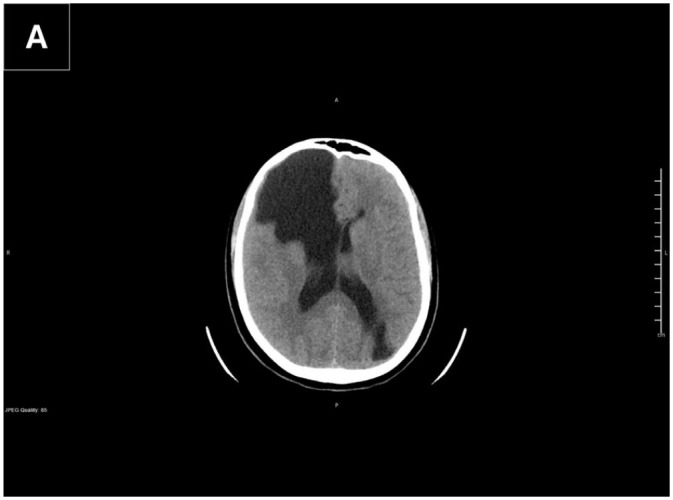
(a) Axial non-contrast CT of the head demonstrates a large porencephalic cyst in the right frontal lobe communicating with the right lateral ventricle, associated with ex vacuo ventricular dilatation and chronic calvarial remodeling. A second porencephalic cyst is seen in the left occipital lobe communicating with the occipital horn of the left lateral ventricle. No acute intracranial hemorrhage or significant mass effect is identified. R/L orientation markers are visible. CT: computed tomography.
